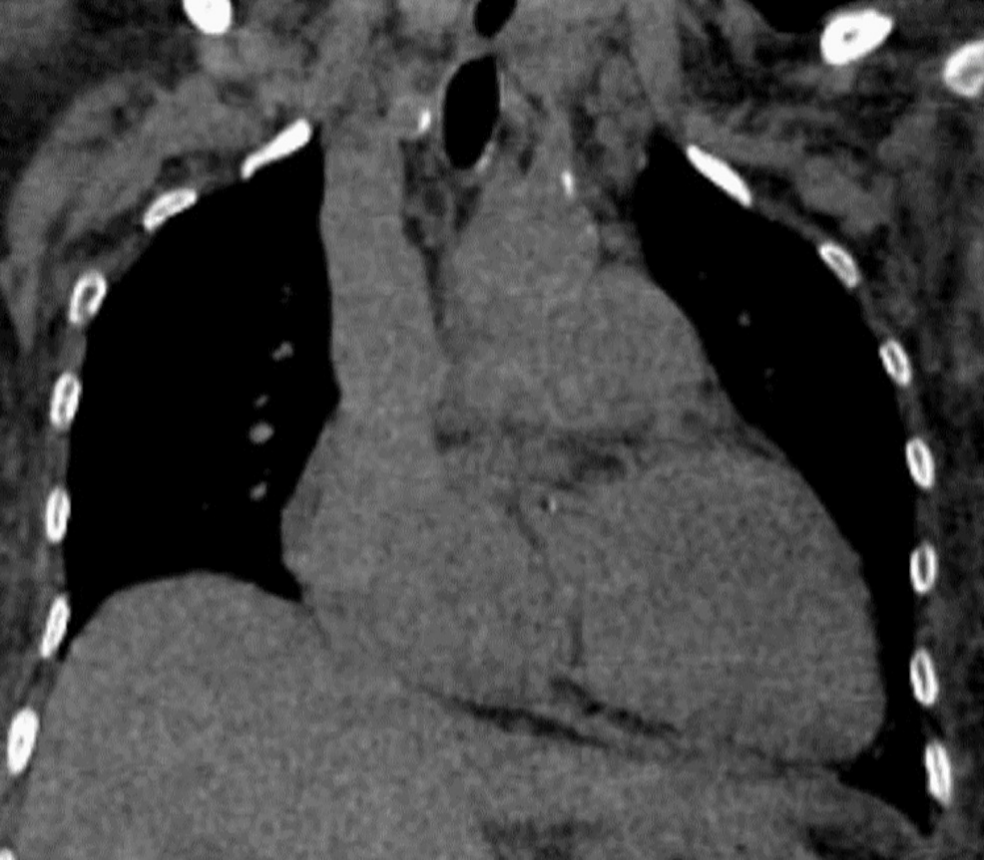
CT scan of the chest in the coronal plane demonstrating cardiomegaly
